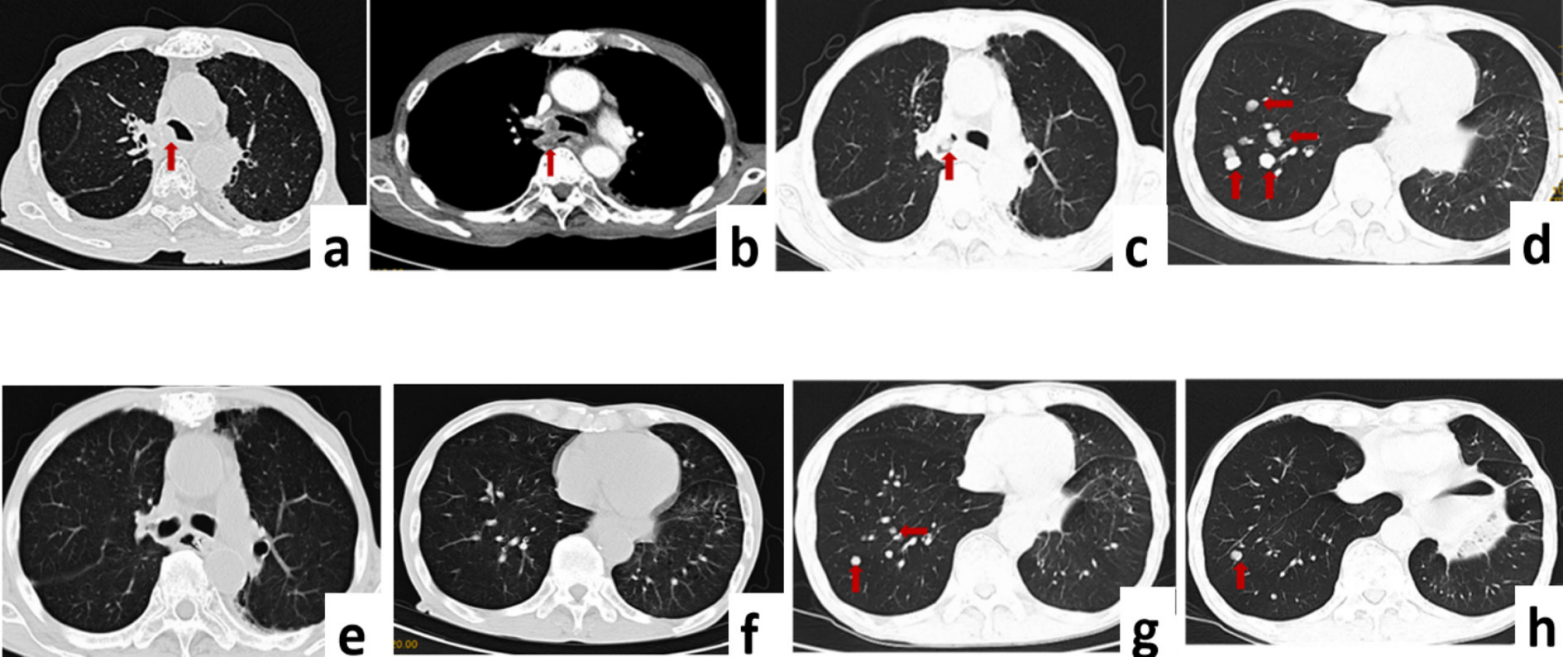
Enhanced CT of the lung. (a, b) Carina and right main bronchus lesions. (c, d) Tumour recurrence in the right main bronchus and multiple metastases in the right lung. (e, f) Complete response of metastatic lesions in the right main bronchus and lung after 6 months of immunotherapy. (g, h) Progression after treatment of lung lesions, new multiple lung metastases.
